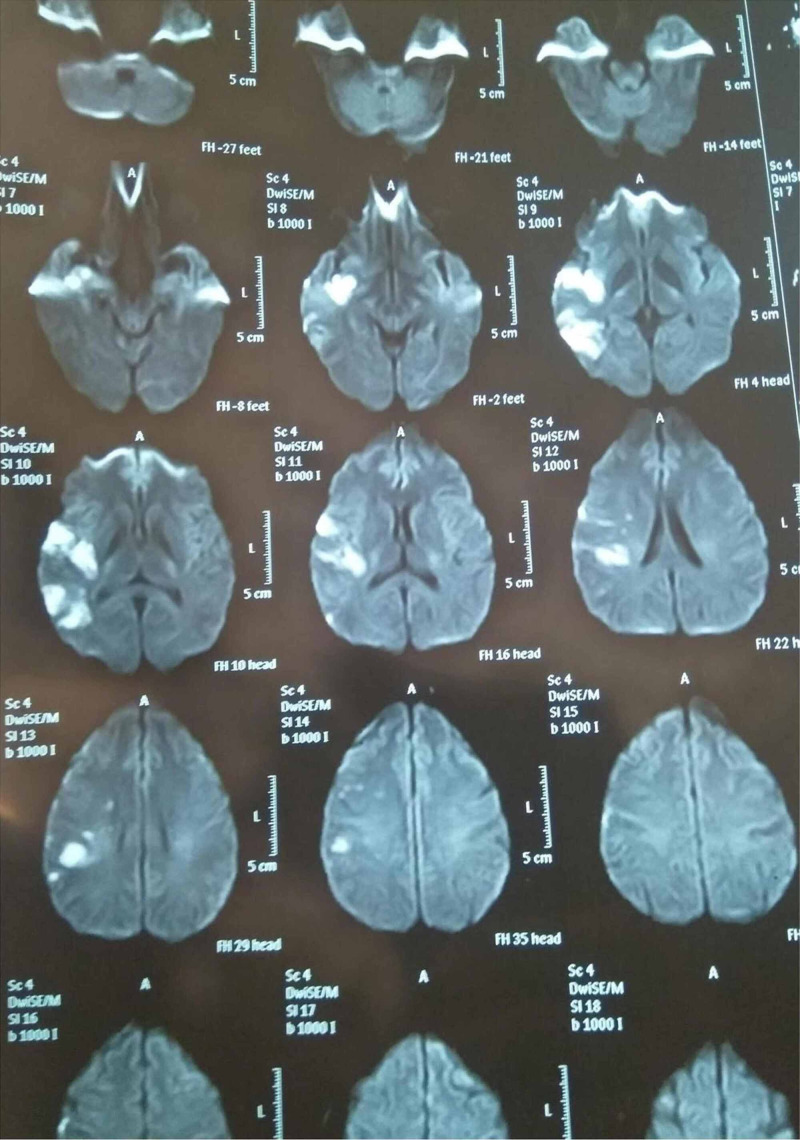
Diffusion Weighted MRI on day of admission.
